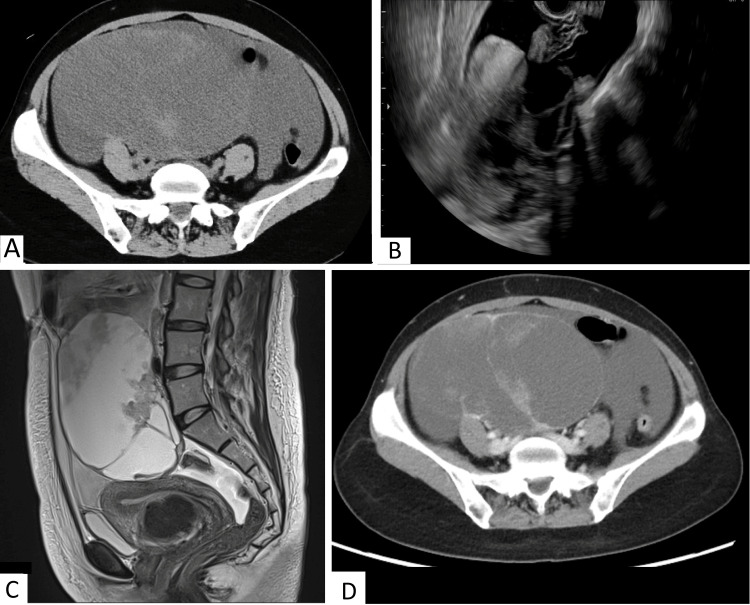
Preoperative imaging findings in a 37-year-old woman with elevated serum hCG showing a right ovarian mass and massive ascites. (A) Non-contrast computed tomography at the referring facility showed a large right adnexal mass and intraperitoneal fluid accumulation. (B) Transvaginal ultrasonography demonstrated a multilocular cystic mass arising from the right adnexa. (C) Pelvic magnetic resonance imaging demonstrated a 15-cm multilocular cystic mass arising from the right ovary with focal diffusion restriction. (D) Contrast-enhanced computed tomography confirmed the large right ovarian mass with massive ascites and showed no lymphadenopathy or distant metastases. hCG: human chorionic gonadotropin
